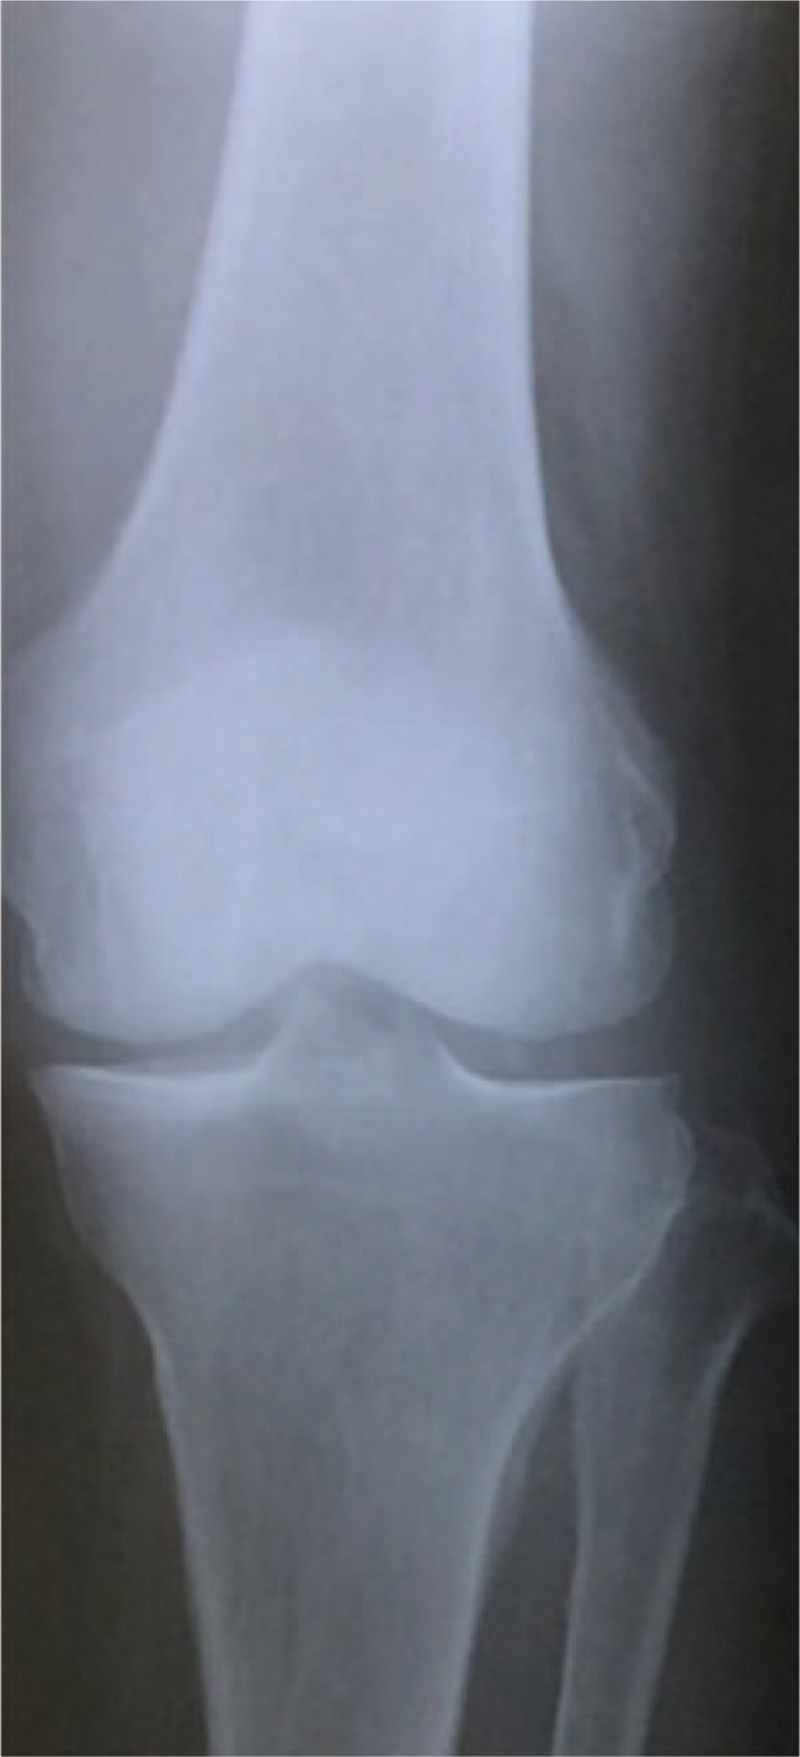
Case 1 in the initial phase. Plain X-ray shows no osteoarthritic lesion.
